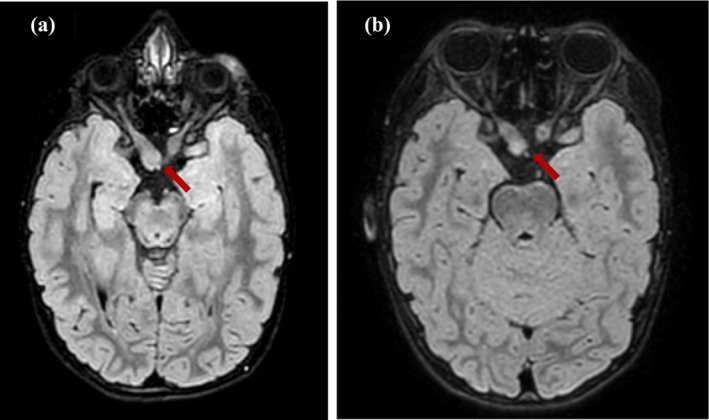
Axial FLAIR MRI (a) before therapy and (b) 1 year and 5 months into selumetinib treatment showed radiological stability (no size increase) despite significant visual improvement. As indicated by the arrow, there are no significant differences at the optic nerve level.
